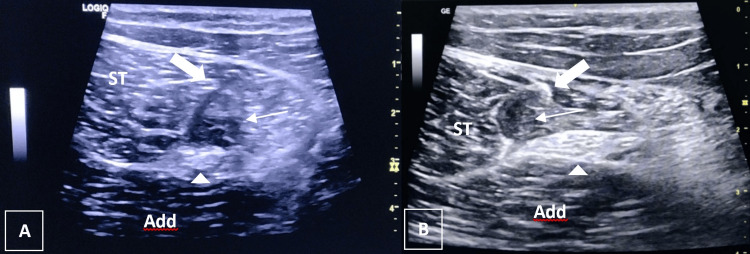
Ultrasound images of the presented case, before treatment (A) and after treatment (B). (A) Transverse scan of the proximal third of the posterior thigh with an immature myotendinous rupture with <25% of the cross-sectional area, adapted BAMIC Ib. (B) Transverse scan of the proximal third of the posterior thigh reveals advanced cicatrization. The thick arrow indicates the common tendon of the ST and biceps femoris long head, while the thin arrow points to the rupture, and the arrow-head represents the sciatic nerve. ST: semitendinosus; Add: adductor magnus; BAMIC Ib: British Association of Muscle Injury Classification Ib
